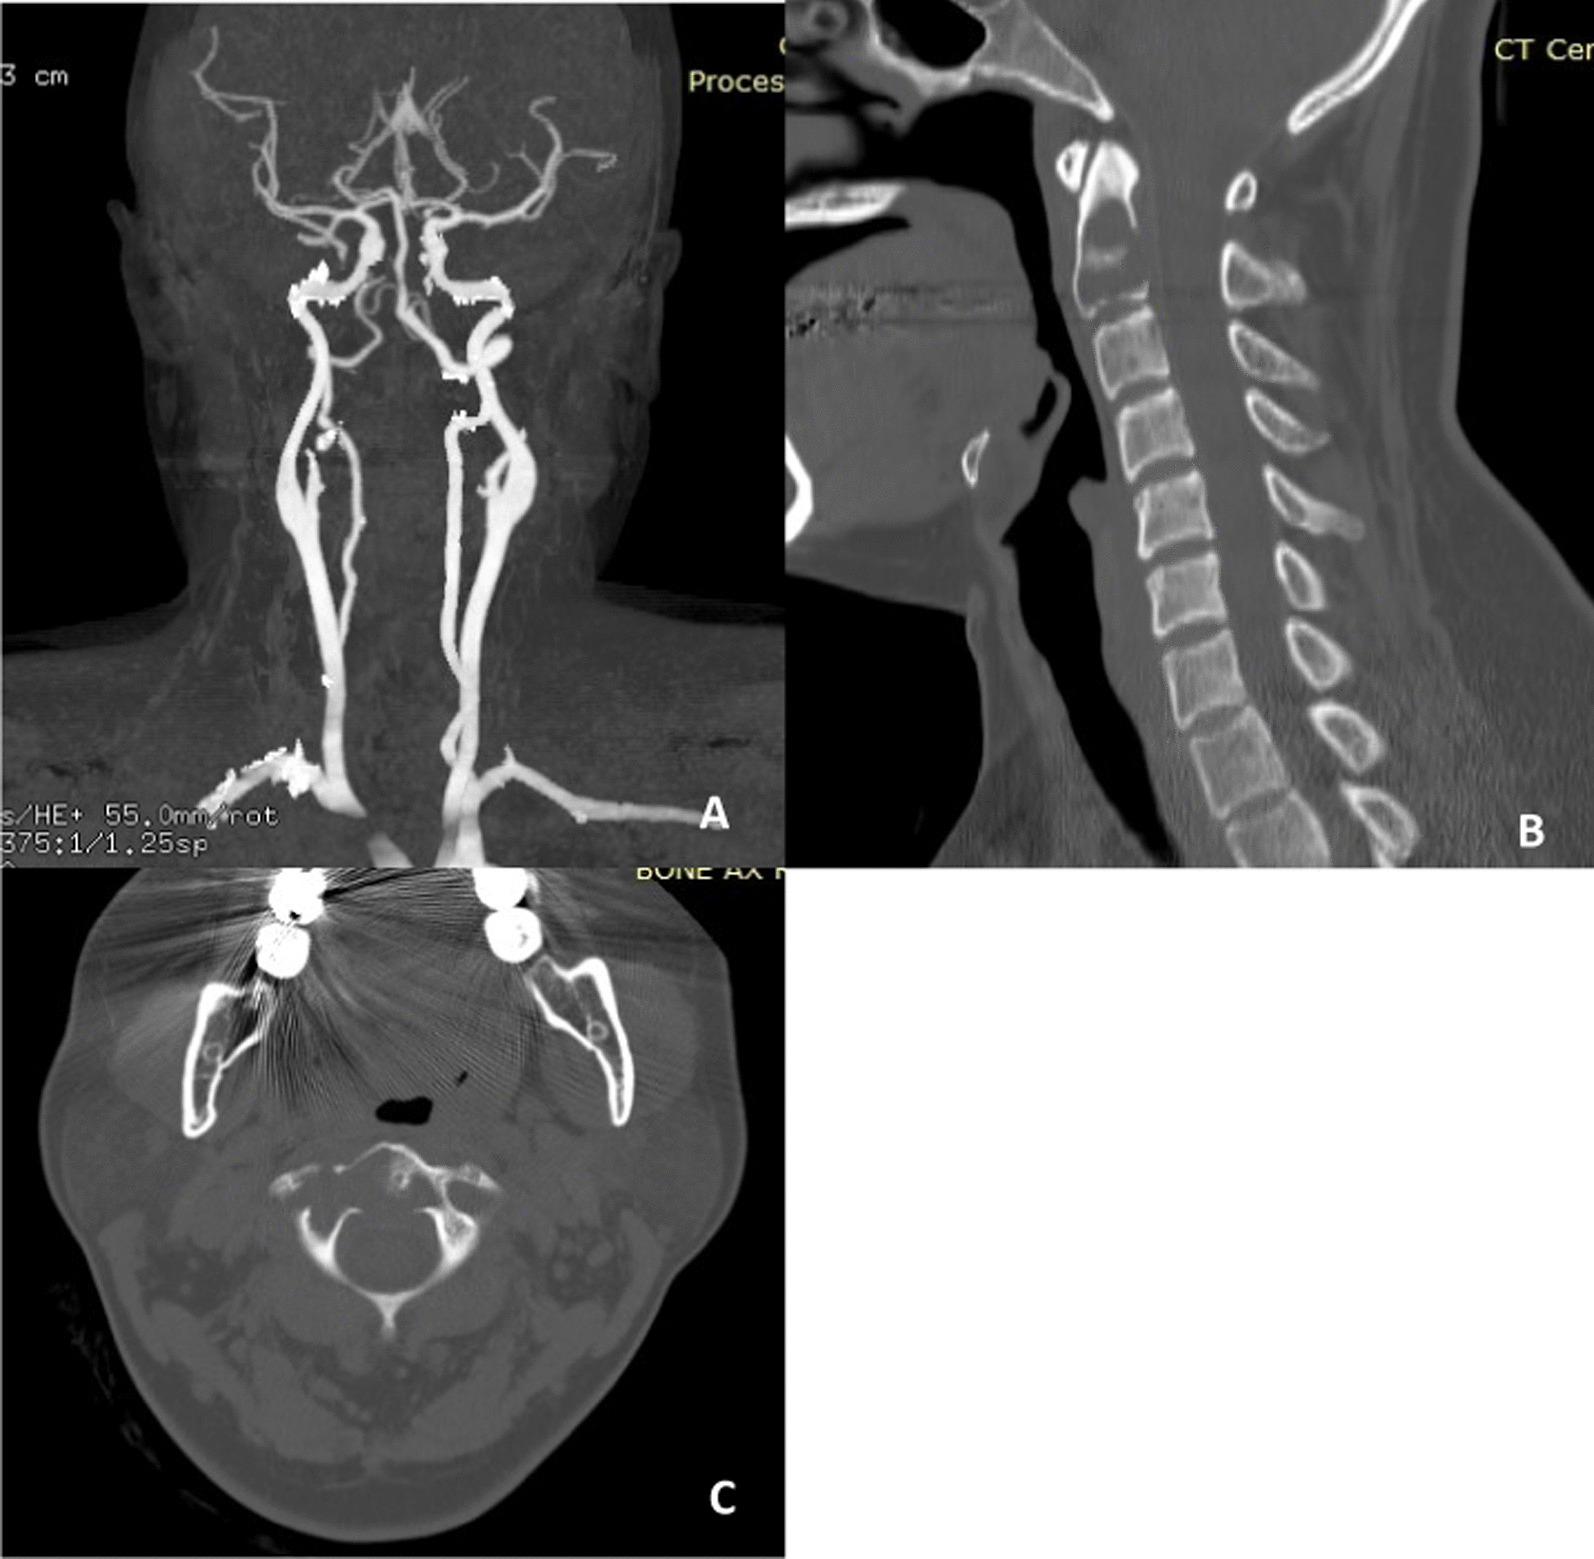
Computed tomography angiography (A) revealed no abnormalities of the bilateral vertebral arteries and a right-side prominence. Sagittal (B) and axial (C) computed tomography of the cervical spine showed a lytic lesion at the body of C2 without sclerotic calcification
